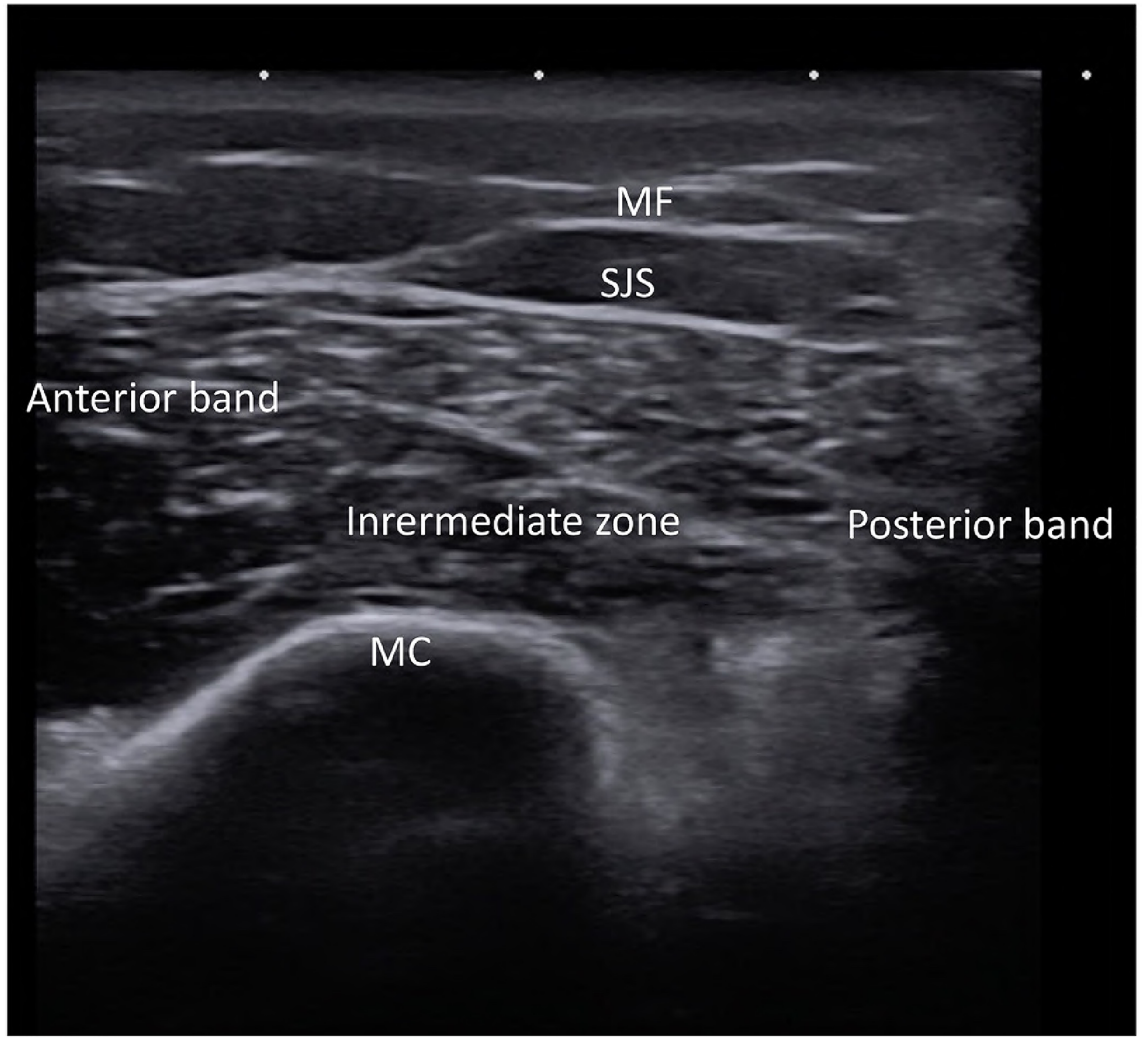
Ultrasound of right temporomandibular joint of healthy subject showing normal architecture of mandibular condyle (MC), mandibular fossa (MF), superior joint space (SJS) and the three sections of the meniscus (anterior, intermediate and posterior).
